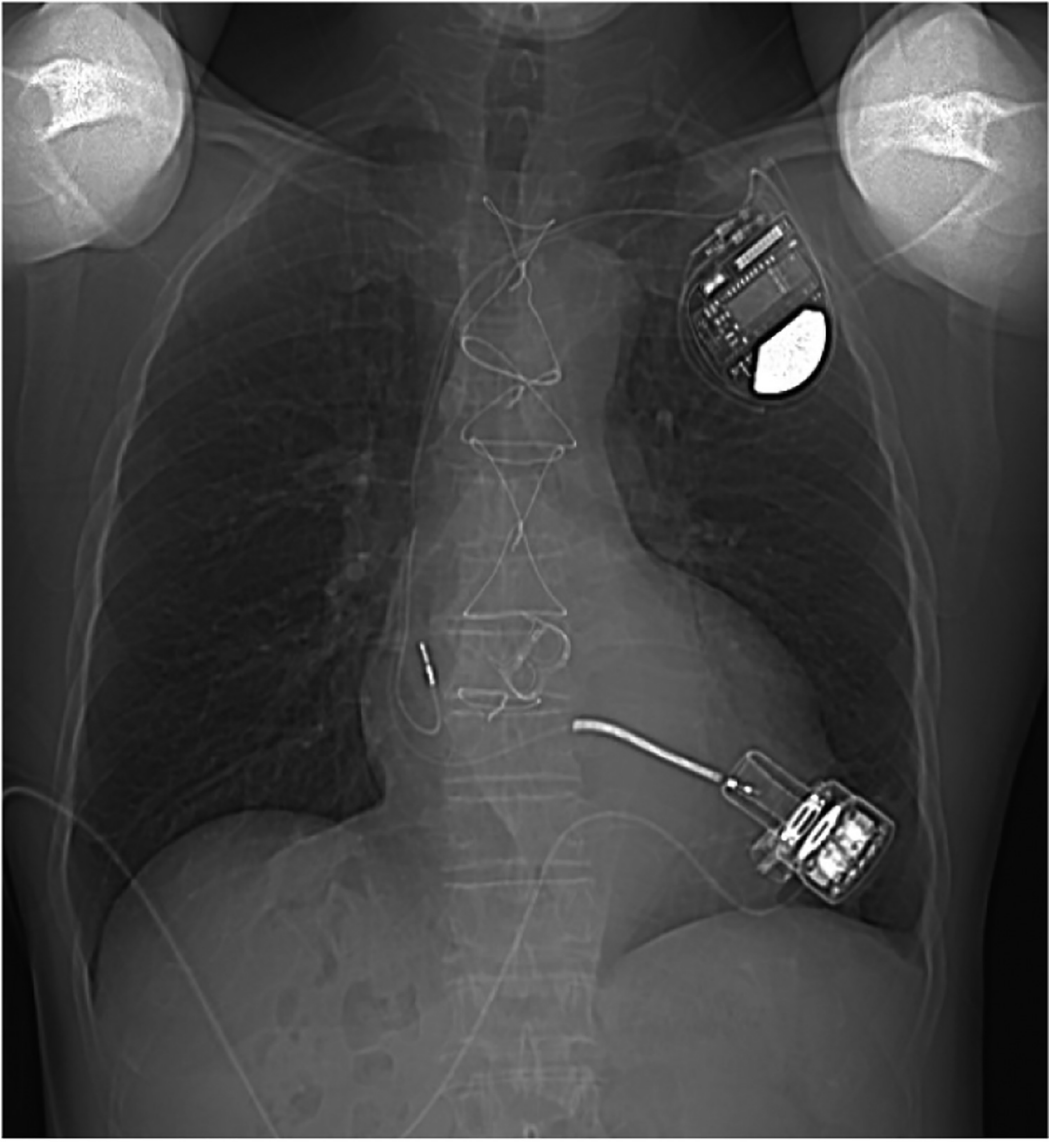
A chest x-ray was performed 1 year after discharge.
